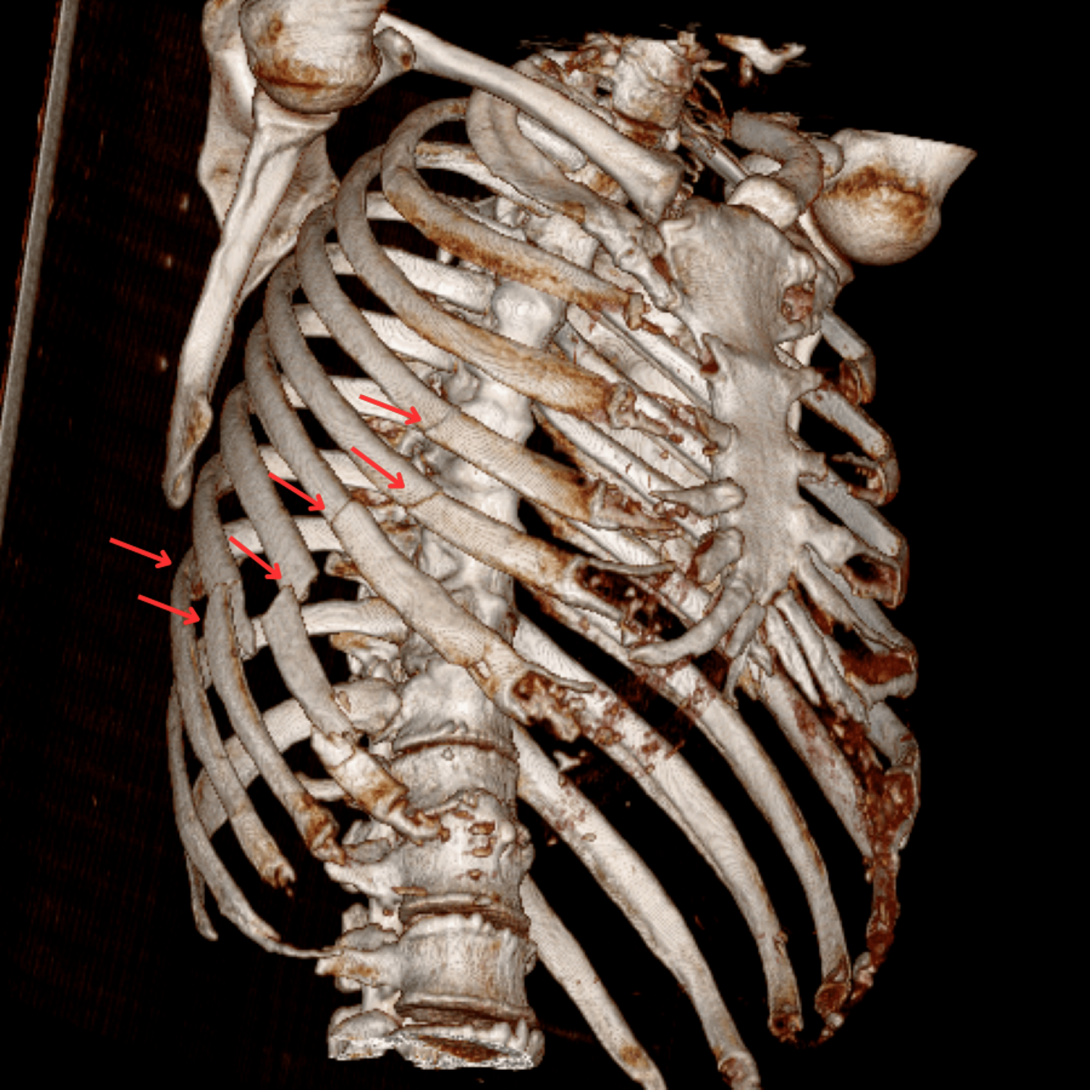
3D reconstruction on CT scan: fracture of 4th to 9th ribs (arrow).
